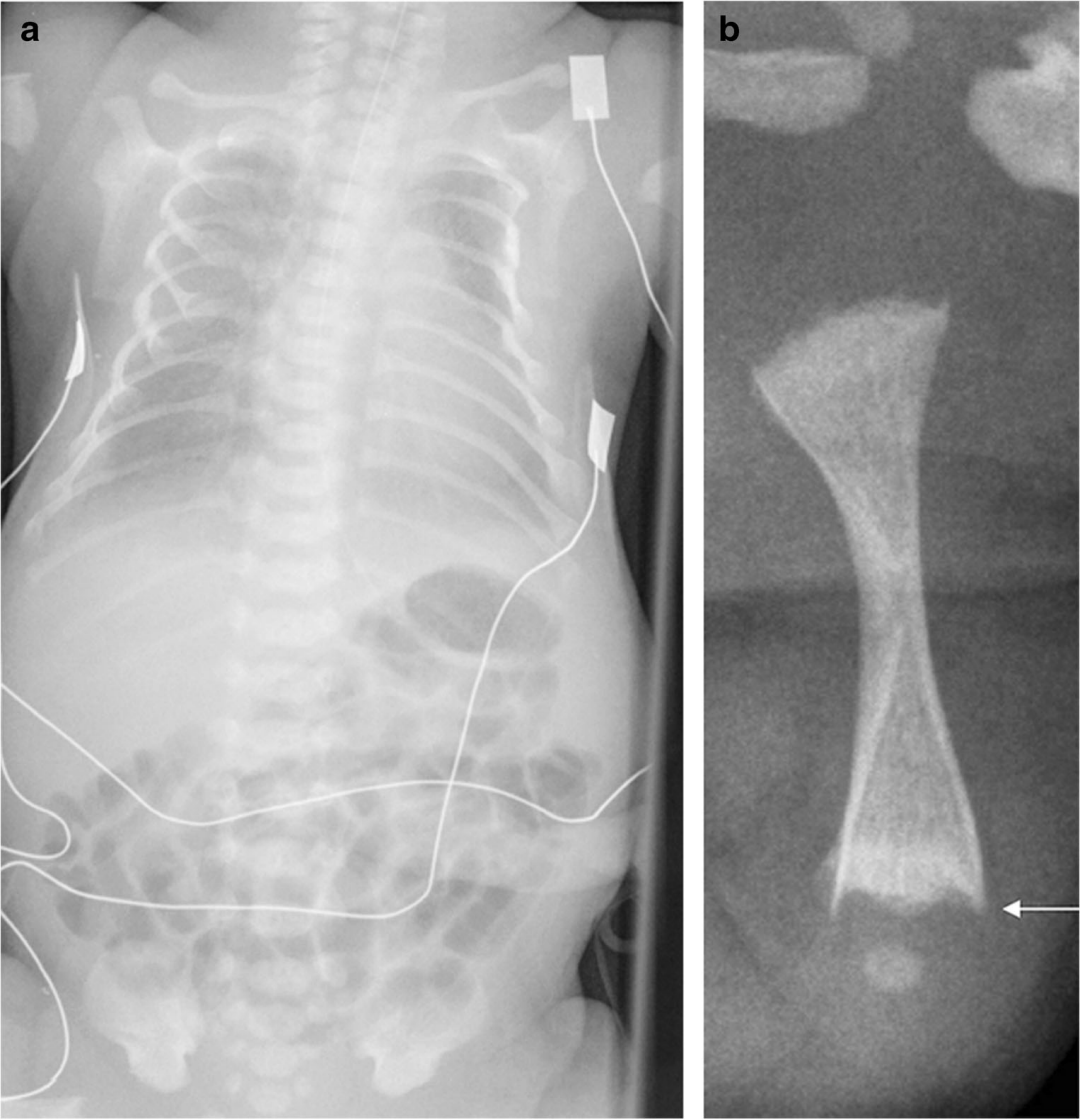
Radiographic features of a girl with osteogenesis imperfecta type V*.*a,b Anteroposterior radiographs show slender, deformed ribs of the chest and abdomen on day 1 (a) and spurred, sclerotic and flared metaphyses (*arrow*) of the left femur at 6 weeks of age (b). [Images reproduced with permission from Radcliffe Publishing [38], page 365, case 1, images 1c and 1e]
